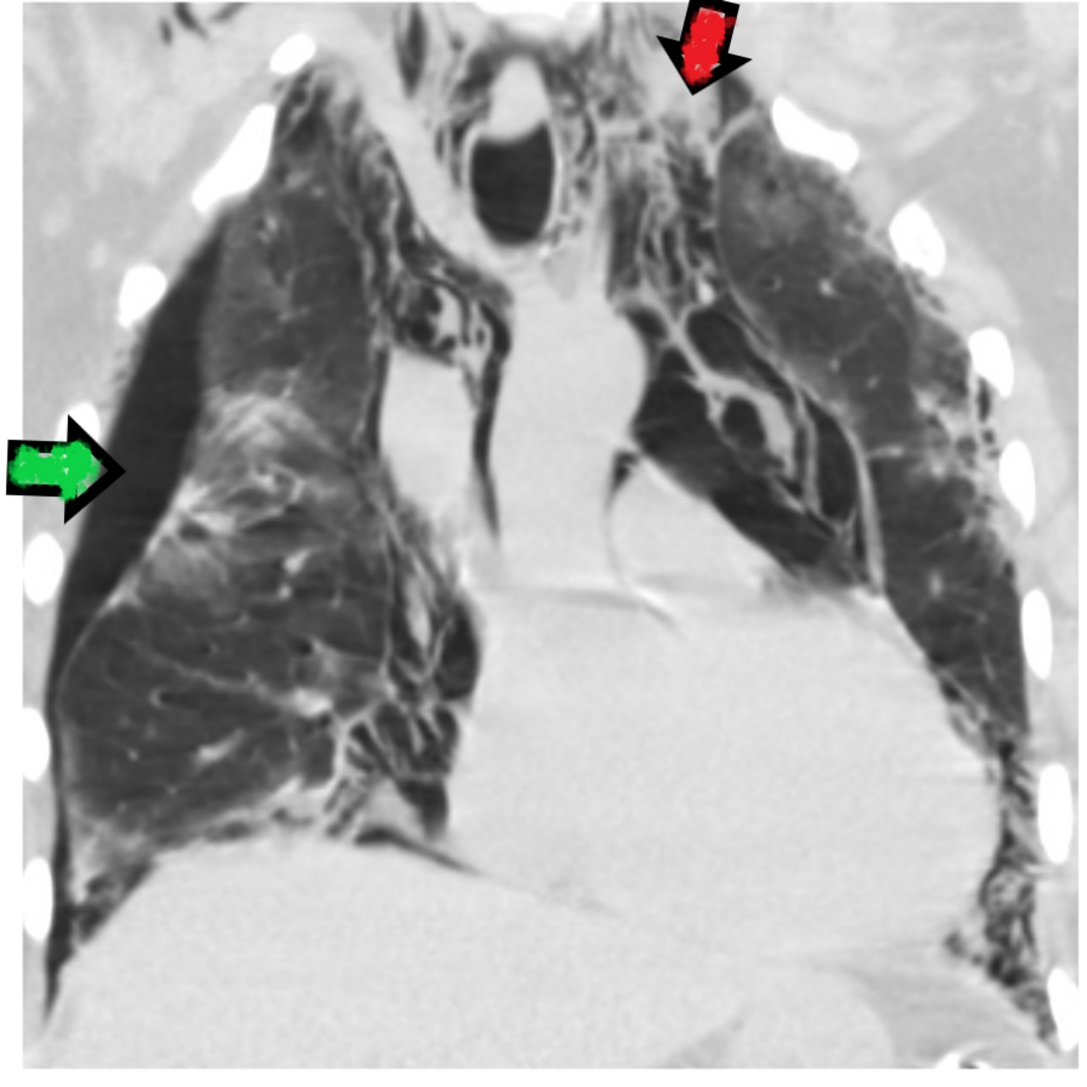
Computed tomographic image of chest on day 8 of admission. Green arrow represents pneumothorax and red arrow represents pneumomediastinum.
